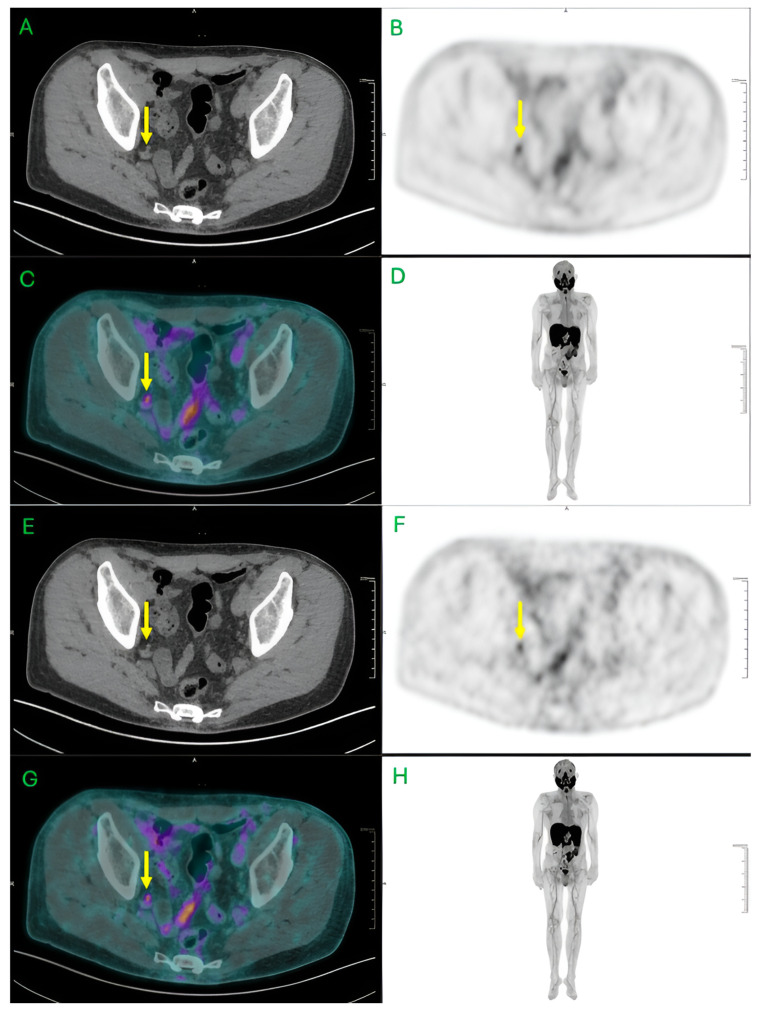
Preservation of high sensitivity using fast acquisition protocol on uEXPLORER Total Body PET/CT. LAFOV Total Body PET/CT (194 cm-uEXPLORER, United Imaging, Shangai, China) with 18F-Piflufolastat (190 MBq) in the restaging process of prostate cancer patient with biochemical relapse after prostatectomy (PSA 0.63 ng/mL). (A–D) represent axial PET/CT images and Maximum Intensity Projection (MIP) from standard acquisition protocol (1 bed of 8 min); Yellow Arrow on a 4 mm right internal iliac nodal disease with mild activity (SUVmax 2.23; Tumor Background Ratio—TBR = 7.43). Images (E–H) show similar qualitative PET/CT images from a post-processing reconstruction just for 1 min acquisition (SUVmax 2.2; TBR = 8.14). Images provided by Nuclear Medicine Centre, San Pietro Fatebenefratelli Hospital, Rome, Italy.
